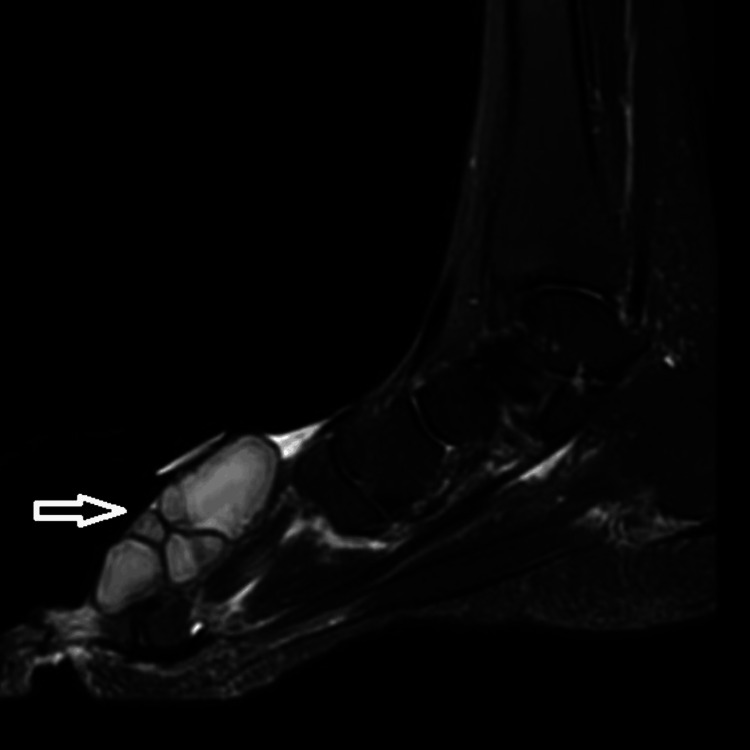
MRI of the right foot A large multilocular lobulated septated cystic mass size of 6 x 6 cm at the first-second intermetatarsal interval between metatarsal heads (arrow) suspicious for large ganglion cyst vs synovial sarcoma.
